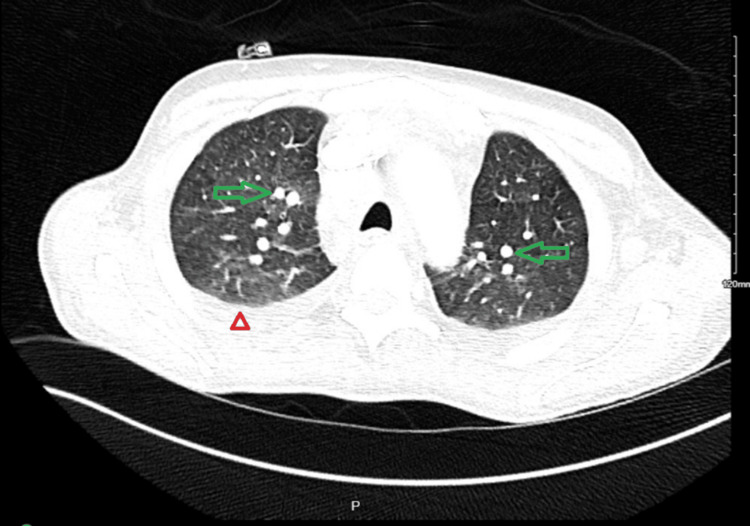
CT angio chest (axial view) Green arrows: lymph nodes; Red arrowhead: pleural effusion CT, Computerized Tomography
